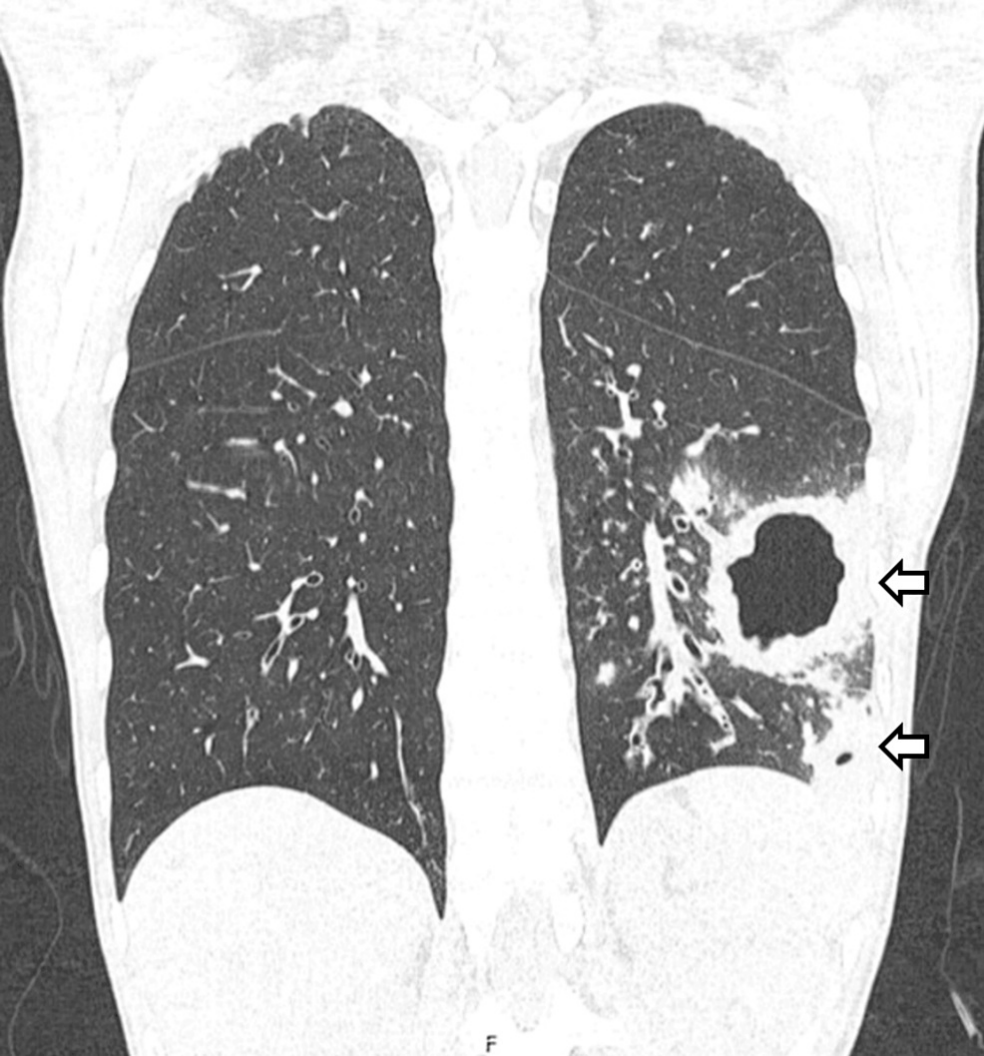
CT scan image (coronal view) one month after hospital discharge CT scan showing three necrotizing masses in the left lung (besides other nodular formations in both lungs) one month after completion of antibiogram-guided antibiotic therapy. CT, computed tomography
